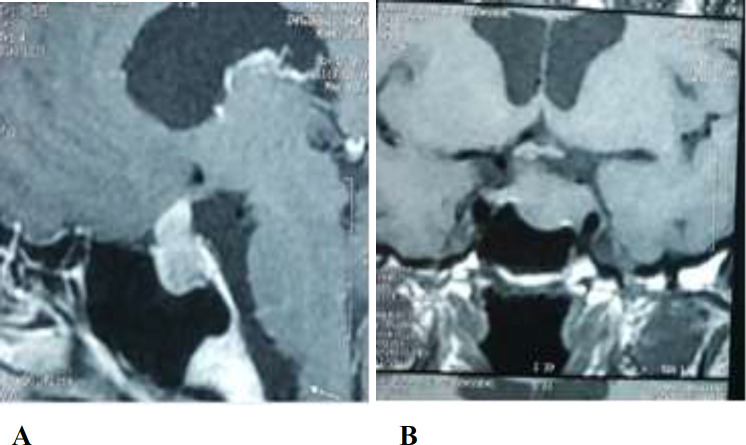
Preoperative T1 imaging (MRI) of the sellar mass (sagittal A, coronal B, view) demonstrating pituitary macro adenoma
